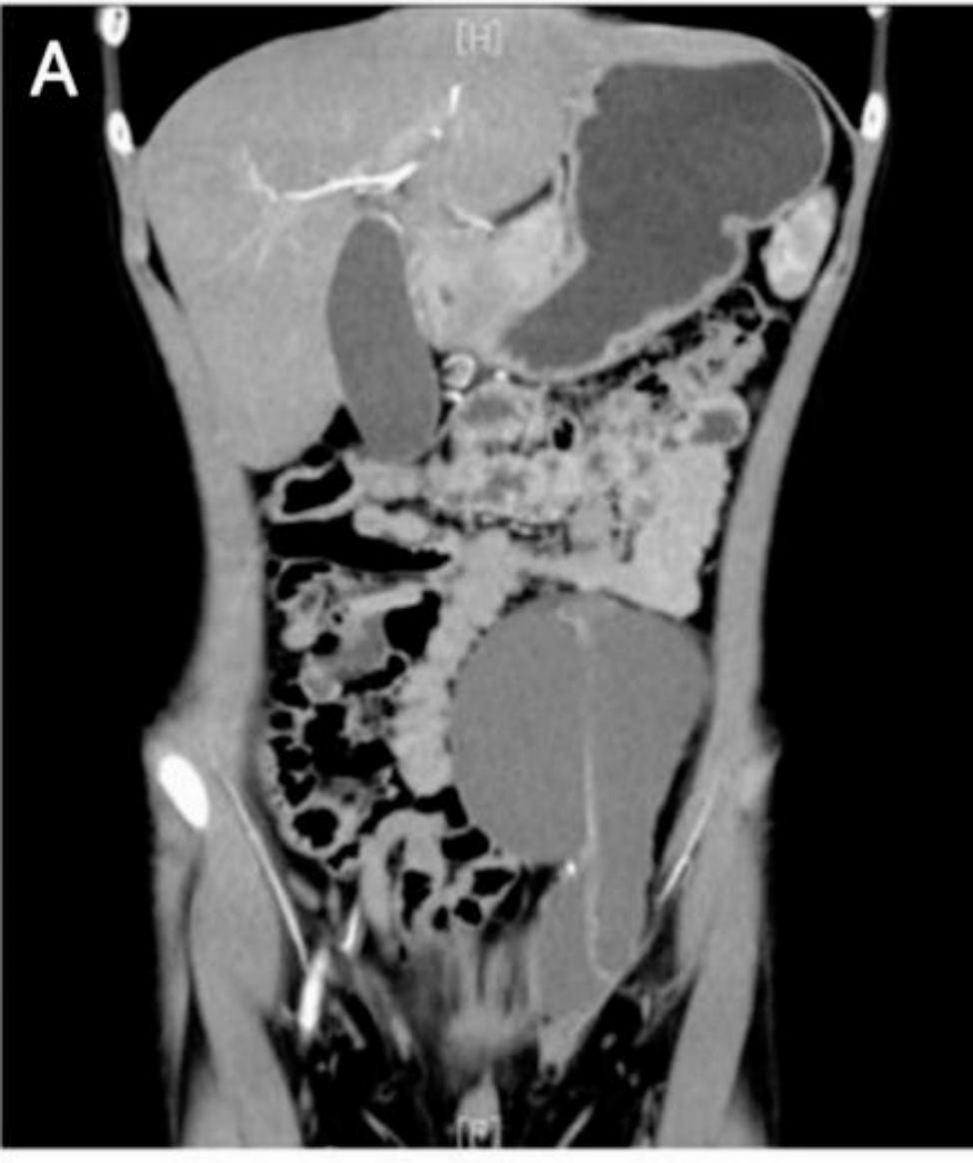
Contrast-enhanced computed tomography scan in Case 9 suggested the possibility of lymphangioma
